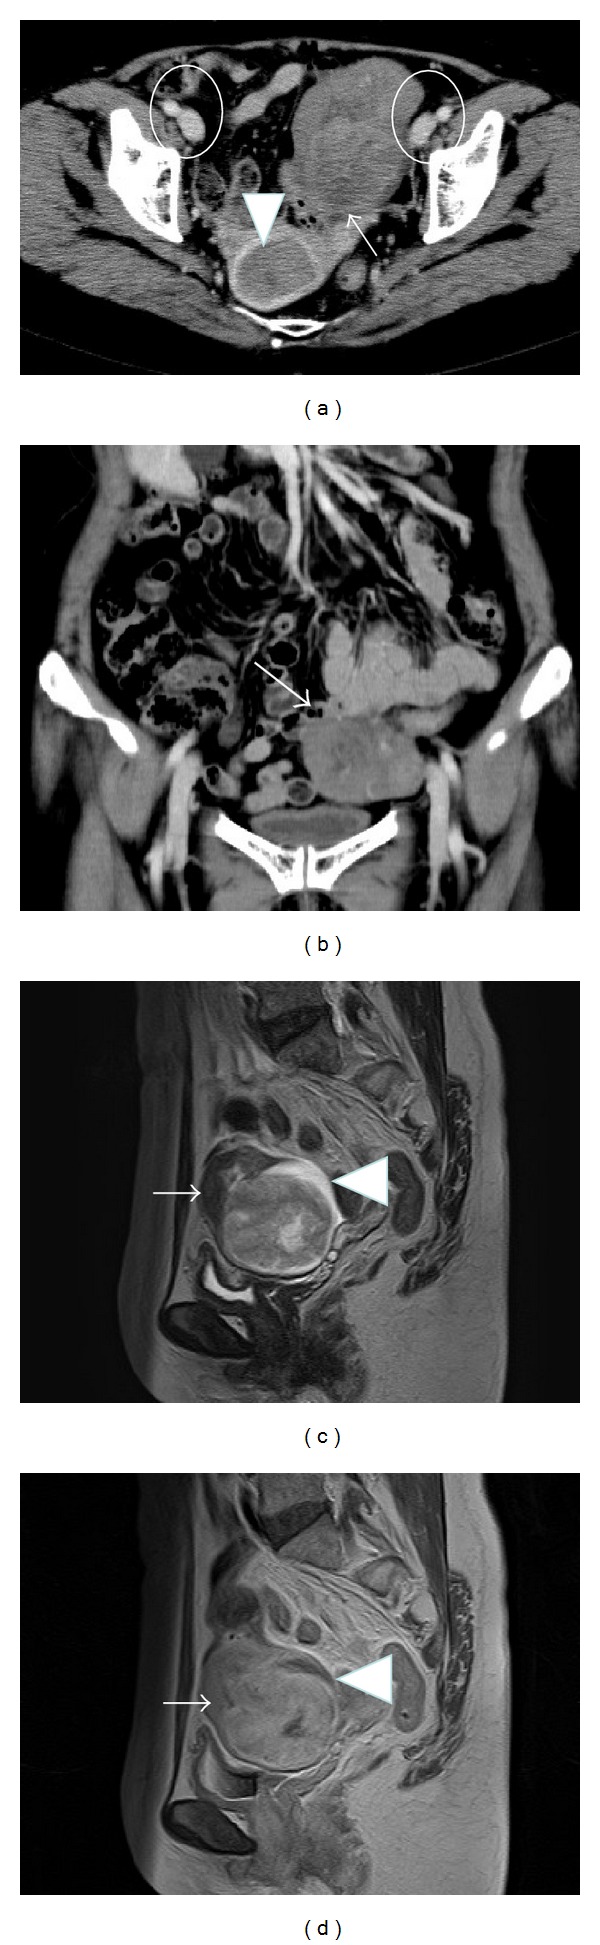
Axial (a) and coronal (b) computed tomography and sagittal magnetic resonance imaging (c, d). (a) Two tumors were detected in both the uterus (triangle) and the peritoneal cavity (arrow). The bilateral ovaries (circle) could be observed. (b) The tumor in the peritoneal cavity originated from the omentum (arrow). (c) The tumor in the peritoneal cavity heterogeneously exhibited an isointense-to-hyperintense signal on T2-weighed images. (d) The tumor was prominently enhanced on contrast-enhanced T1-weighted images. The flow void from the omentum to the tumor (arrow) and ascites (triangle) could also be detected.
